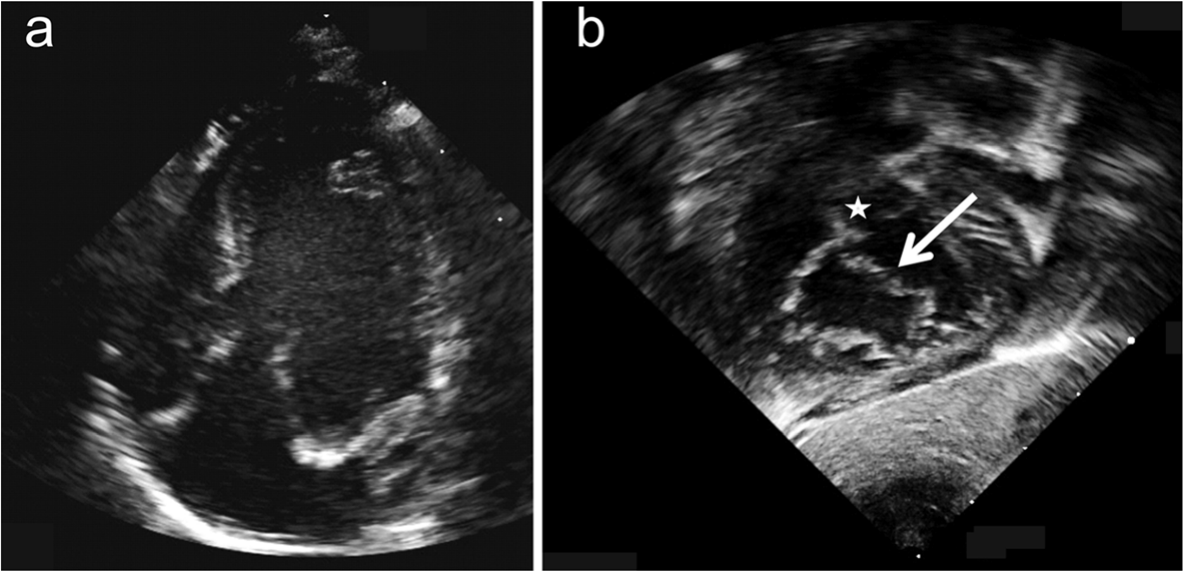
Apical 4-chamber view in patient 1 shows absent left atrioventricular connection, UBC, hypoplastic right ventricle and a solitary atrioventricular valve straddling a large inlet VSD (a). The subcostal LAO-view (b) shows the morphology of a common atrioventricular valve with a superior bridging leaflet (arrow) inserting to the right of the ventricular septum (asterix)
